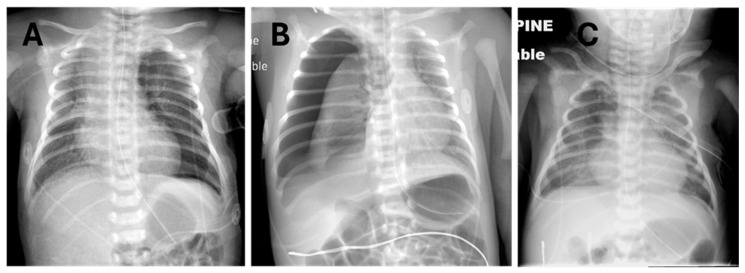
Prototypical examples of pneumothorax on chest radiographs before surfactant administration via LMA. (A) Left pneumothorax not drained. (B) Right pneumothorax prior to thoracentesis. (C) Left pneumothorax after chest tube drainage with residual bilateral pneumothorax and pneumomediastinum plus emphysema in the neck tissue planes.
